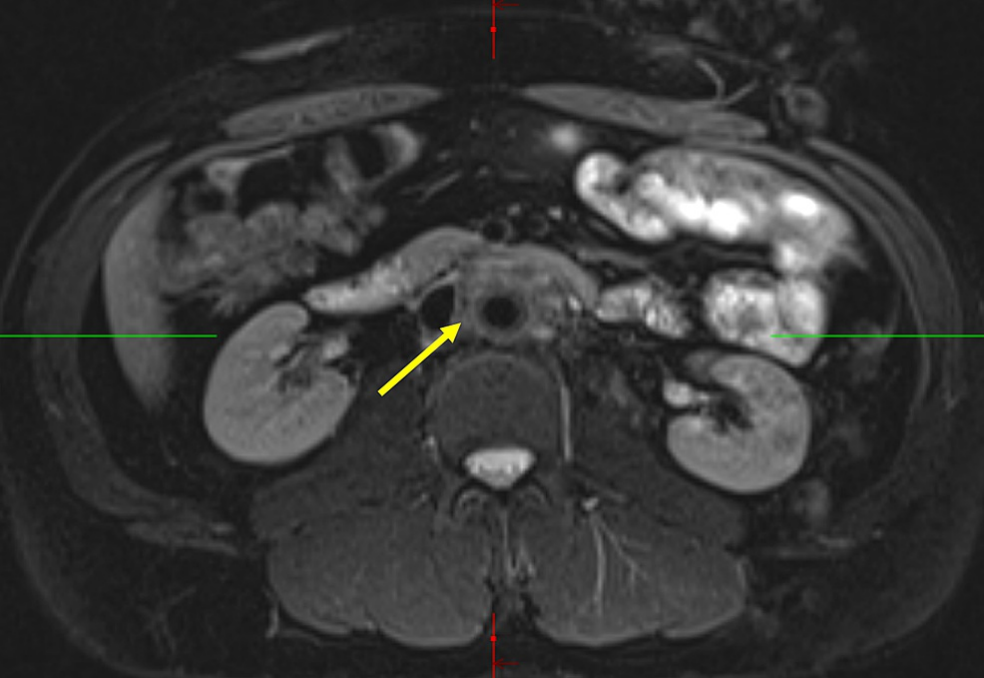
MRI at the time of diagnosis Circumferential soft tissue thickening (arrow) is clearly visible around the infrarenal aorta. The ureters are not retracted into the soft tissue thickening and there is no hydronephrosis.
